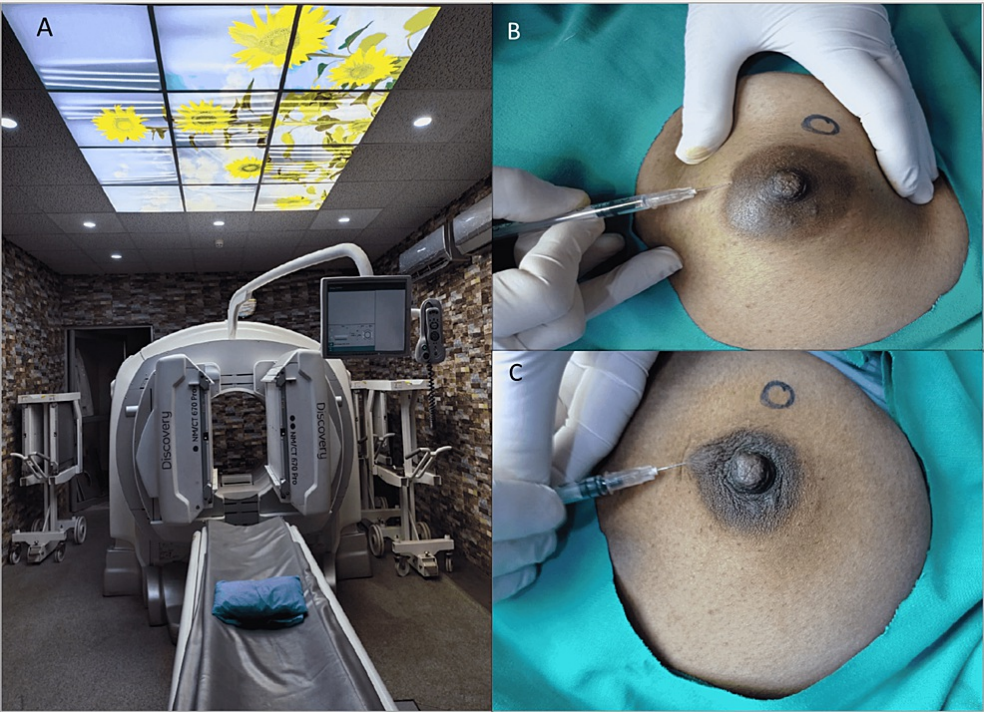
A: SPECT-CT gamma camera; B,C: Peri-areolar injection technique SPECT: single-photon emission computerized tomography
